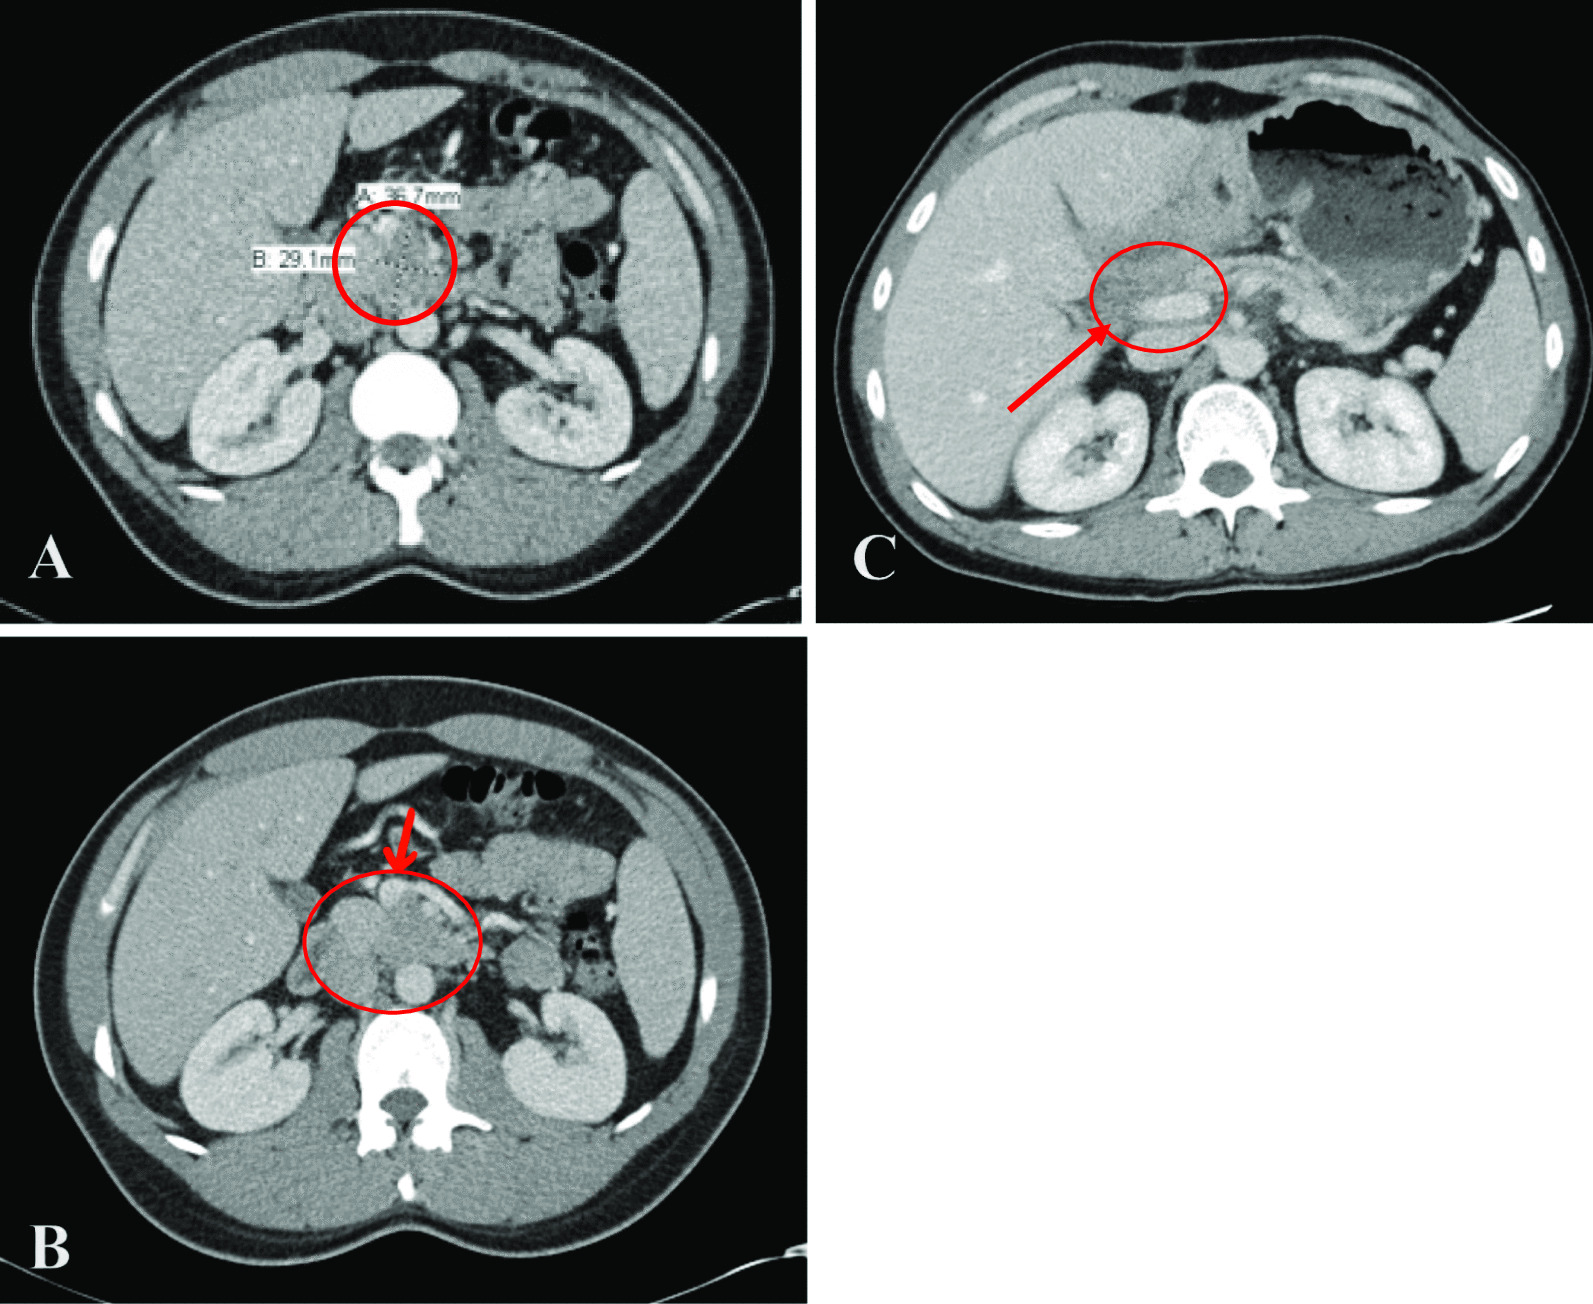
A Pre-operative CT scan of abdomen and pelvis revealing a 3.7 × 2.9 cm ill-defined Pancreatic head /uncinate process mass (red circle). B Pre-operative CT scan of the abdomen and pelvis observing the superior mesenteric vein (SMV, red arrow) prior to resection of tumor (within red circle). C Post-operative CT scan of abdomen and pelvis, showing residual soft tissue (red circle) encasing the superior mesenteric vein (red arrow) but without evidence of definitive disease
